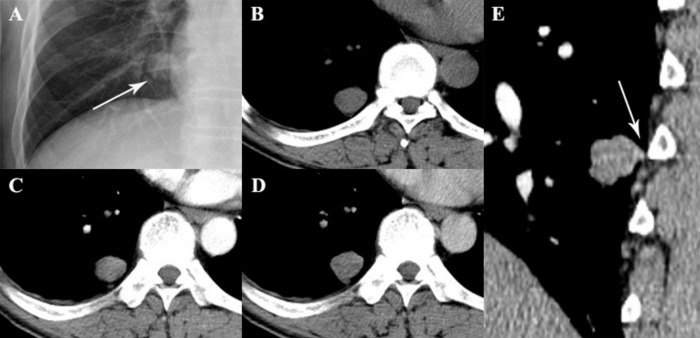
A 49-year-old woman with SFTP. (A,B) Chest radiograph and precontrast CT scan show a small oval mass with well-defined margin in lower right thorax (arrow). (C,D) The mass shows mild enhancement on biphasic postcontrast CT images. (E) A pedicle of the tumor with the adjacent pleura is detected on sagittal MPR image (arrow).
